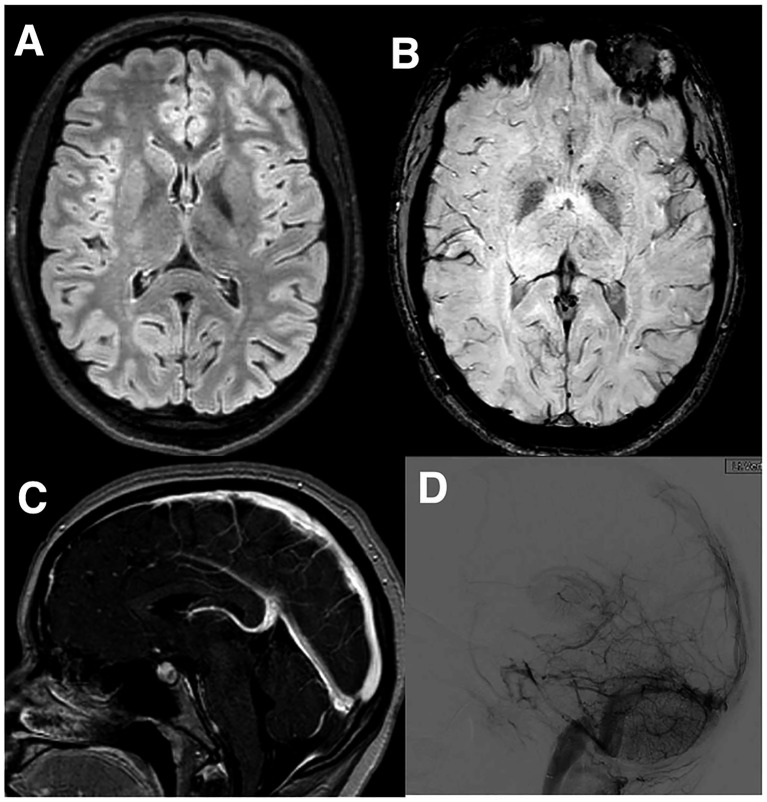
Post-treatment imaging following mechanical thrombectomy and anticoagulation. (A) Axial FLAIR showing interval improvement in bithalamic edema. (B) Susceptibility-weighted imaging demonstrating interval development of a hemorrhagic venous infarct. (C) Sagittal post-contrast imaging demonstrating restored venous opacification. (D) Post-treatment angiography demonstrating improved deep venous outflow.
